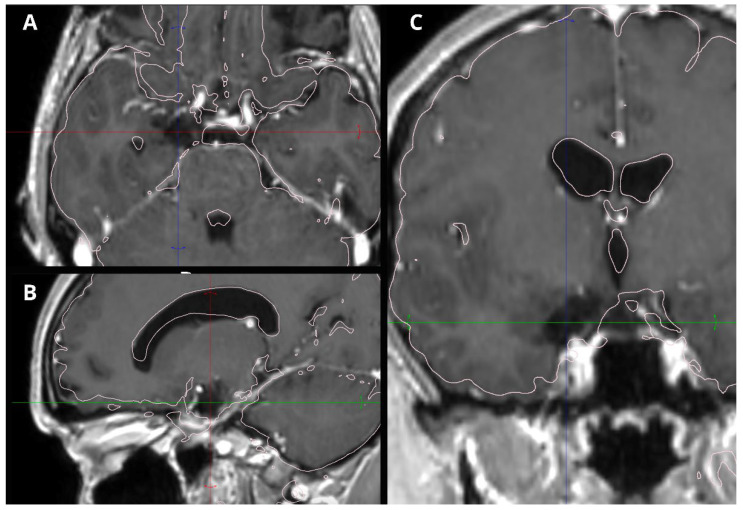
Post-contrast T1-weighted MRI following gross total resection (GTR) of a dysembryoplastic neuroepithelial tumor (DNET) in the right hippocampus via supraorbital craniotomy. (A) Axial scan showing the resection cavity with no residual tumor. (B) Sagittal scan confirming complete tumor removal and preservation of adjacent structures. (C) Coronal scan demonstrating the absence of residual lesion and normal alignment of surrounding anatomy.
